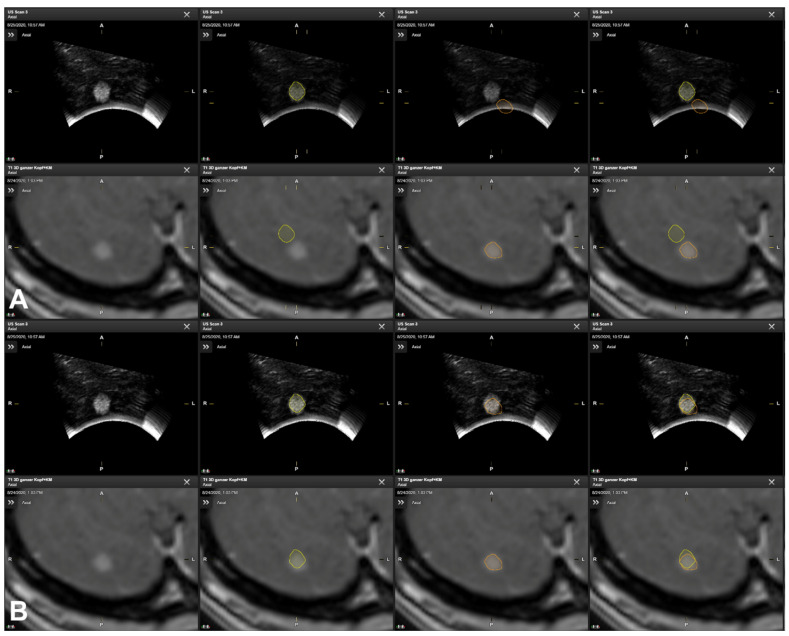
Manual segmentation of tumor outlines based on preoperative MRI data (orange) and navigated iUS data (yellow) showing the spatial mismatch seen in reference array-based co-registration, indicating the need for a navigation update (A), whereas navigation accuracy was increased after rigid image-based co-registration (B), showing a suitable spatial overlap of MRI- and iUS-based tumor outlines.
